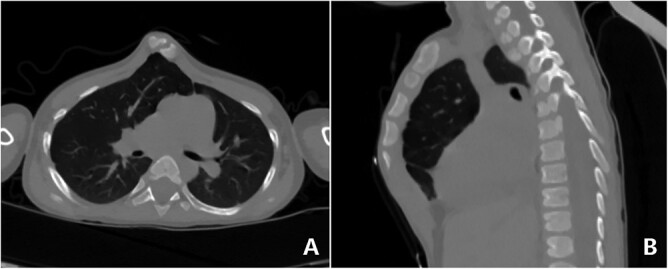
CT examination. (A) Section and (B) sagittal view.
